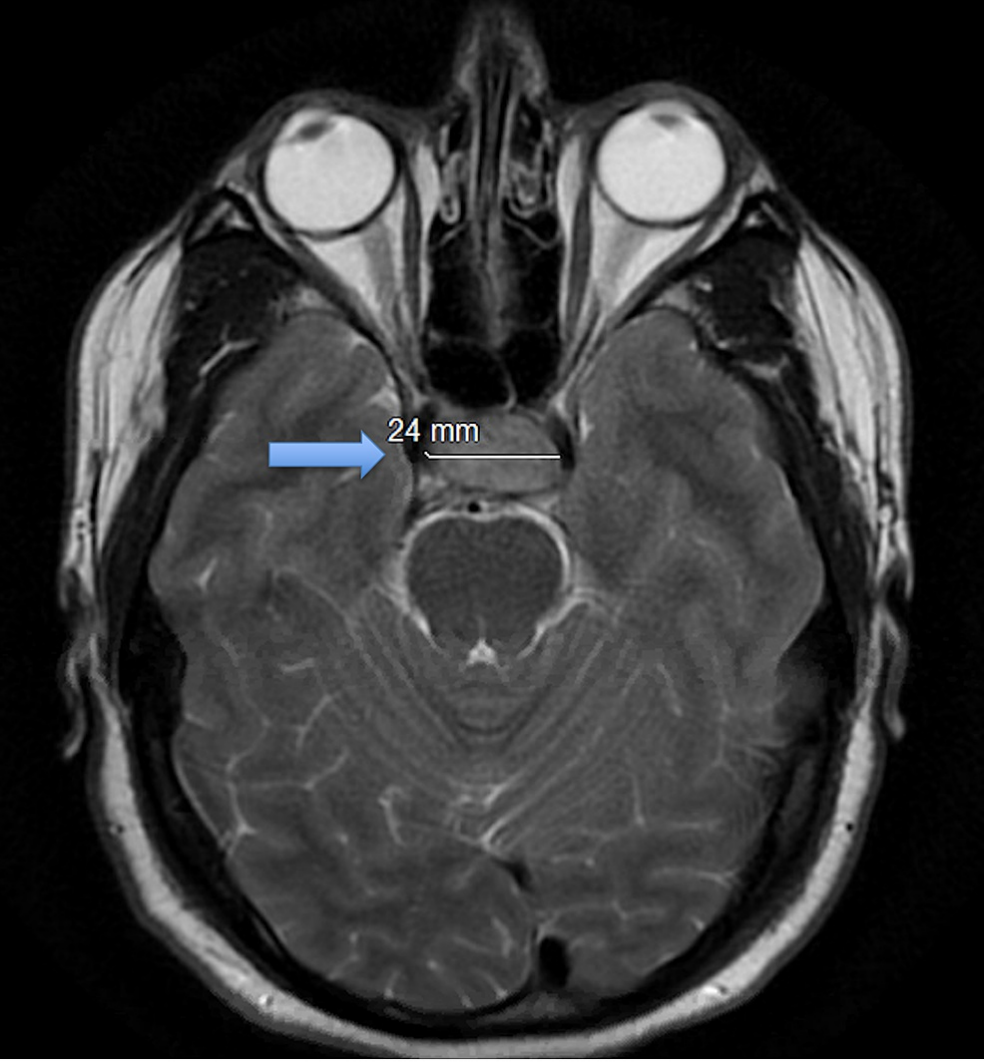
MRI of the brain: axial view demonstrating an area of T1 hyperintensity along the anterior right and lateral aspects of the mass representing blood products consistent with ischemic pituitary apoplexy. MRI: magnetic resonance imaging
